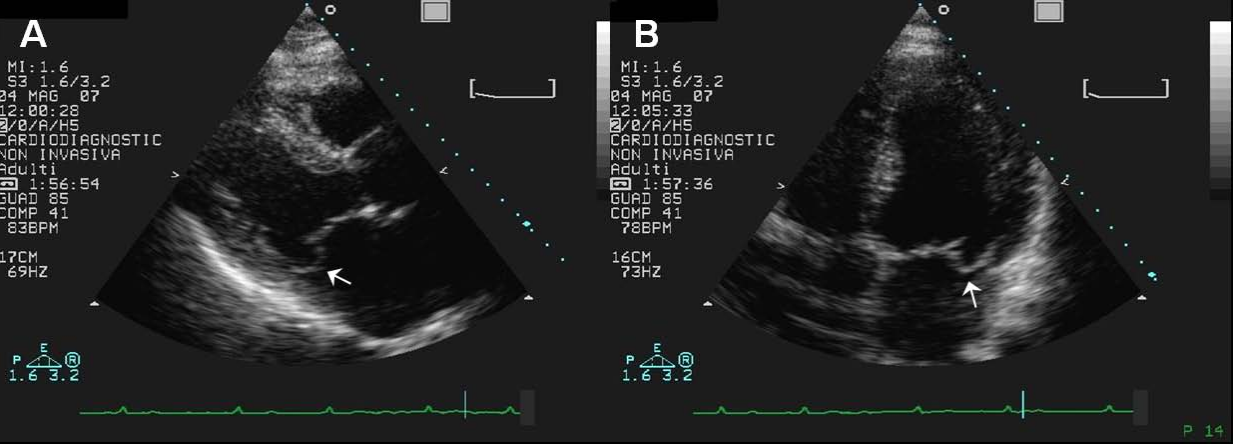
2D-TTE parasternal long-axis (A) and apical four-chamber (B) views: mitral valve prolapse involving P2 and P1 scallops (arrows).
